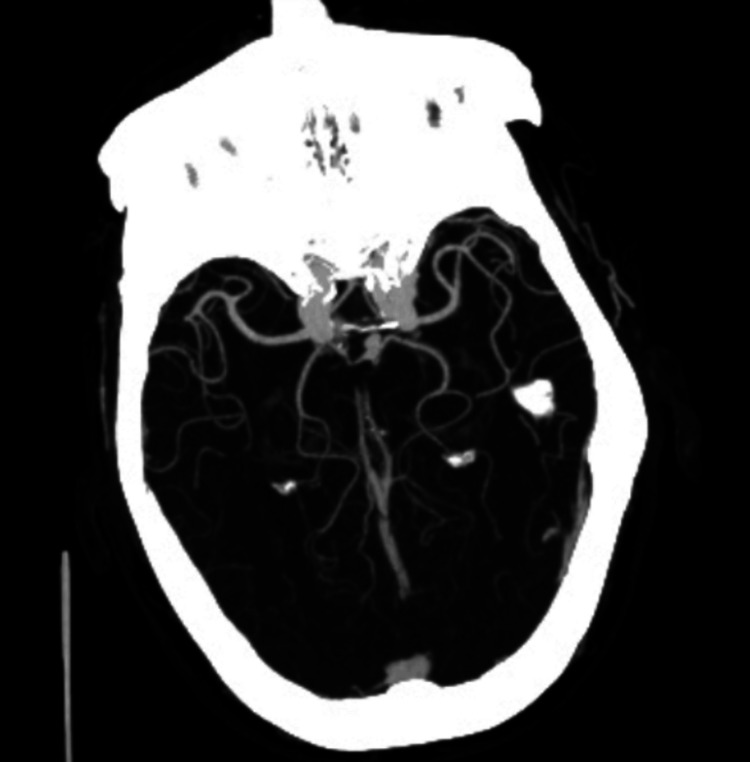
CT angiogram showing patent intracranial arteries
